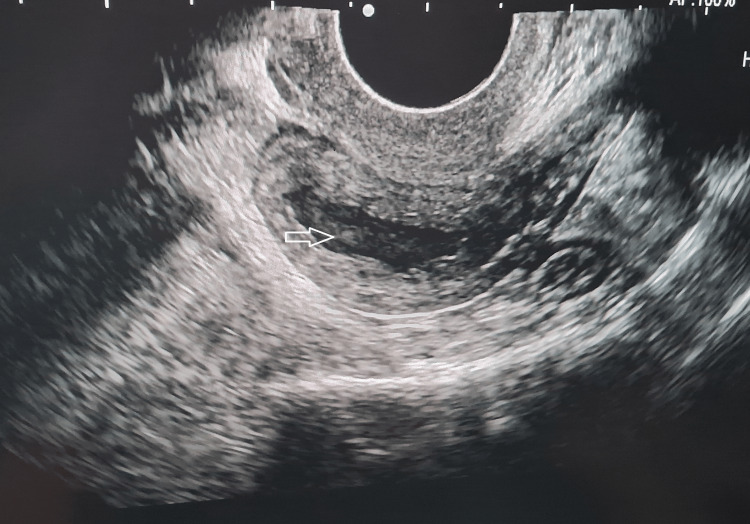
TVS image showing heterogeneous collection with multiple internal echoes within the endometrial cavity. TVS: trans-vaginal sonography
